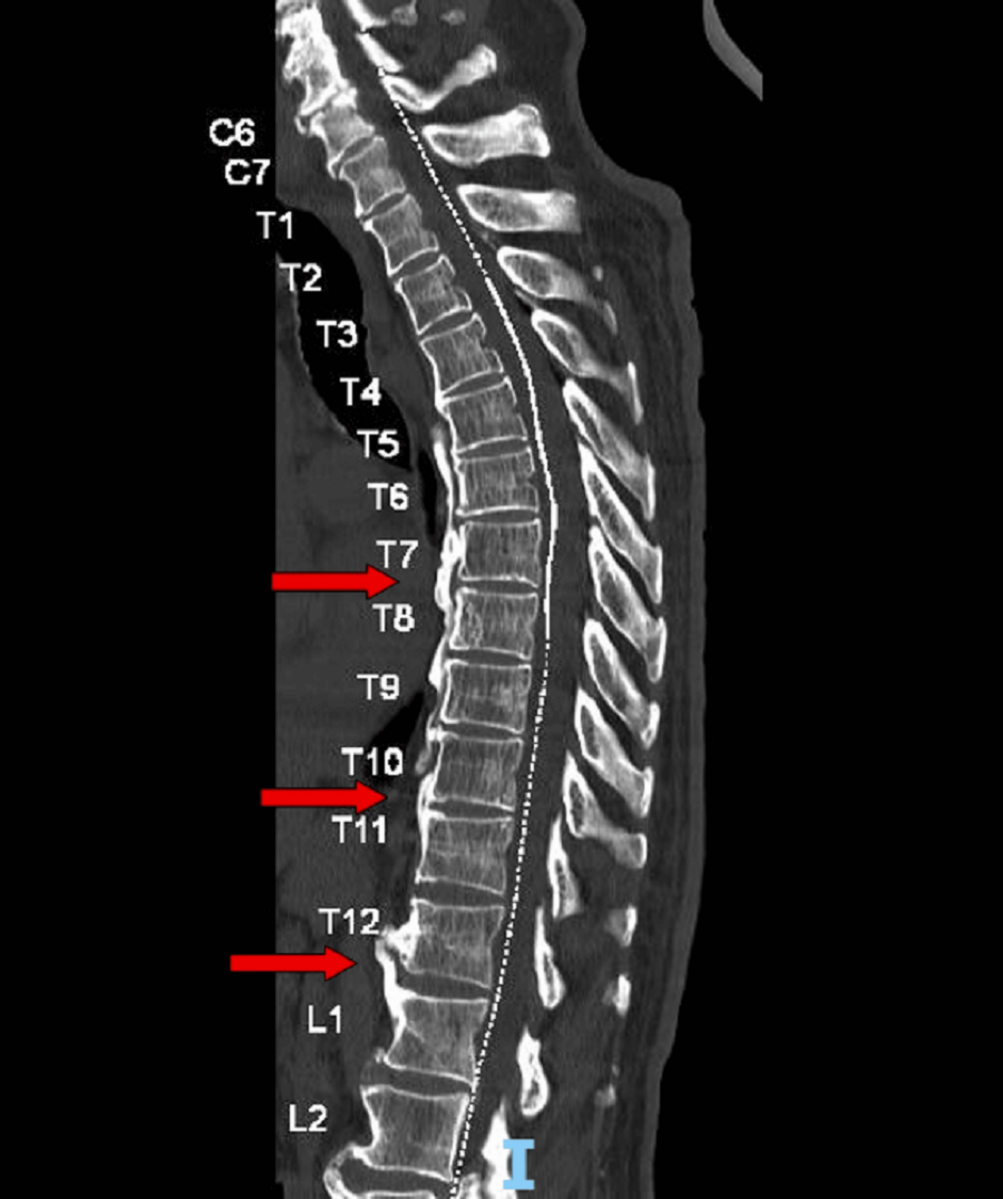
Spinal CT showing findings compatible with DISH. A mild dextroconvex scoliotic alignment is present, without fractures, lytic lesions, or significant canal or foraminal stenosis. Red arrows:  DISH more pronounced in the mid- and lower-thoracic segments, with anterior marginal osteophytes and straightening of the normal curvature. DISH: diffuse idiopathic skeletal hyperostosis
